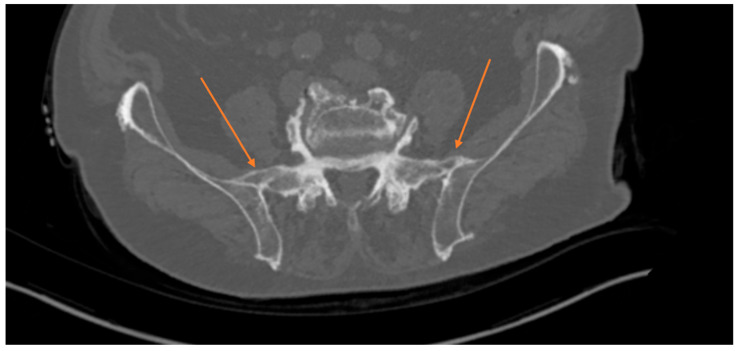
Axial CT of the pelvis showing signs of spontaneous sacroiliac-joint fusion cranial and anterior of the SI joint (arrows) making posterior stabilization of the pelvis unnecessary despite wide resection of the symphyseal region.
